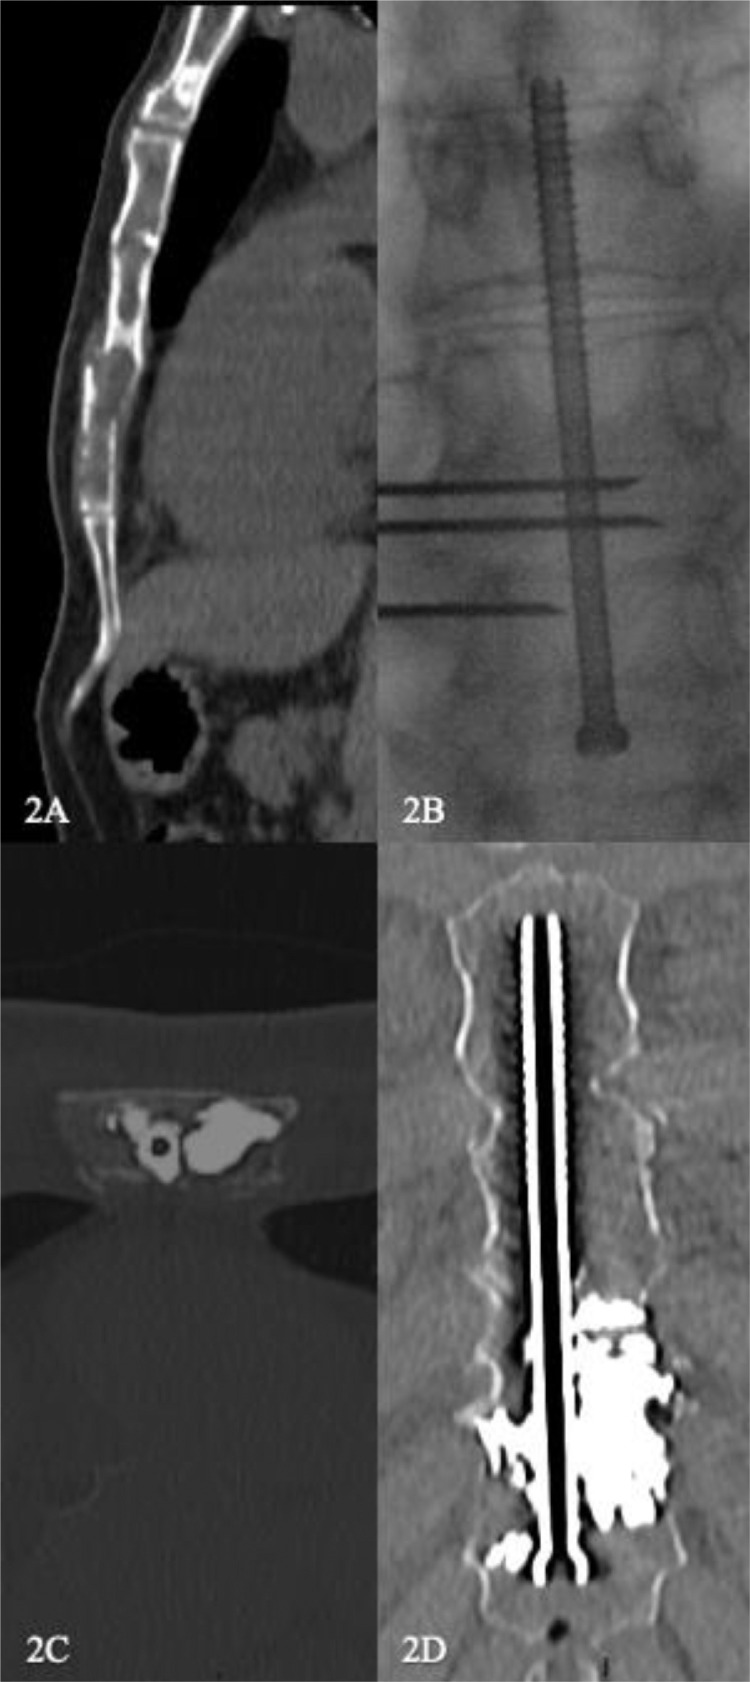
Sixty-eight-year-old man with follicular lymphoma, sternal localization, and a pathologic fracture. (A) Preoperative CT-scan images in sagittal section showing the pathologic fracture with 5 mm cortical disjunction. (B) Frontal fluoroscopy showing location of cementing trocars. (C) CT-scan in axial section showing results after screwing and cementation. (D) CT-scan frontal section showing the result at the end of the procedure, with no leaking of cement, satisfactory fracture stabilization, and no cortical effraction of the screws.
